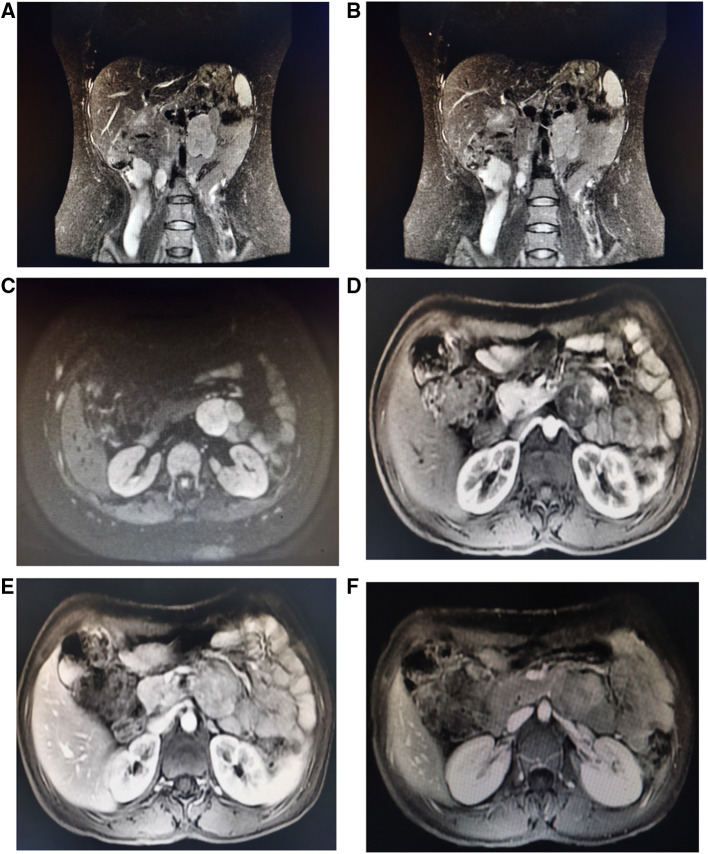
Abdominal MRI shows an irregular retroperitoneal mass under the pancreatic body and the pancreatic uncinate process is pushed forward to the right. The mass had about 3.8 cm × 5.7 cm × 2.8 cm in size, which has unclear boundary with some surrounding intestinal loops (A,B). Coronal T2-weighted image depicts high signal tumor; (C) Transverse Diffusion-weighted image shows obvious high signal tumor; (D–F) enhancement MRI scan on arterial, venous and excretion stages show uneven and obvious enhancement.
